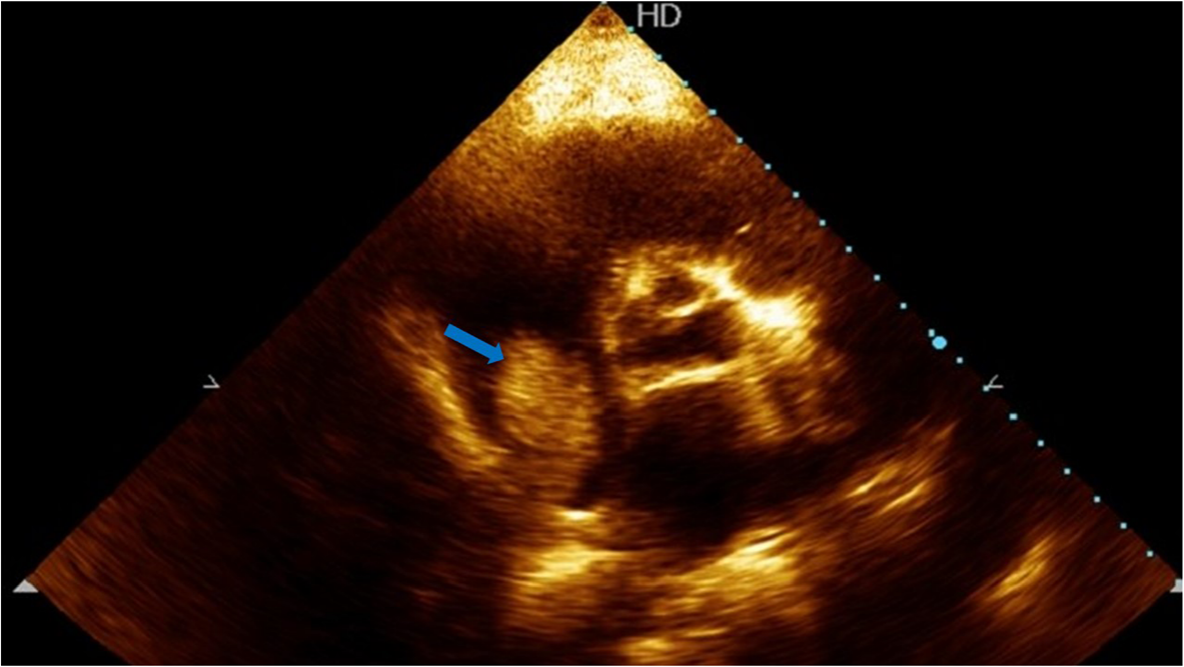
TTE parasternal short-axis view showing a large mass in the right atrium arising from the inferior vena cava marked by a blue arrow
